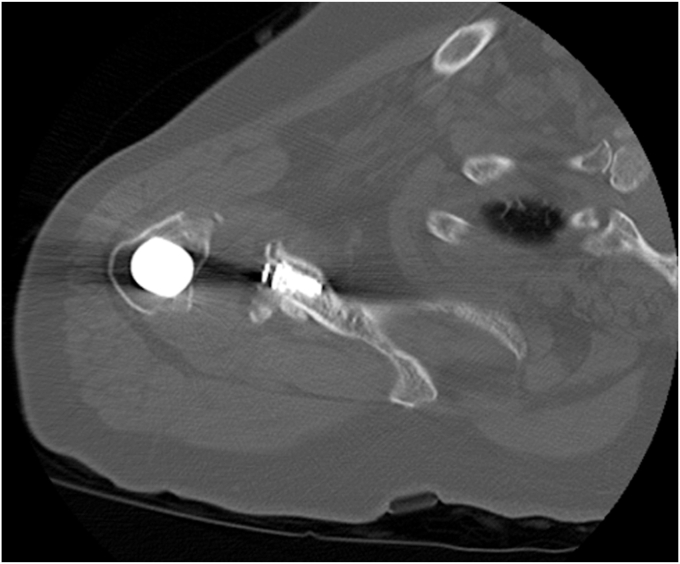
Preoperative CT showing aseptic loosening of prior total shoulder arthroplasty. *CT*, computed tomography.
